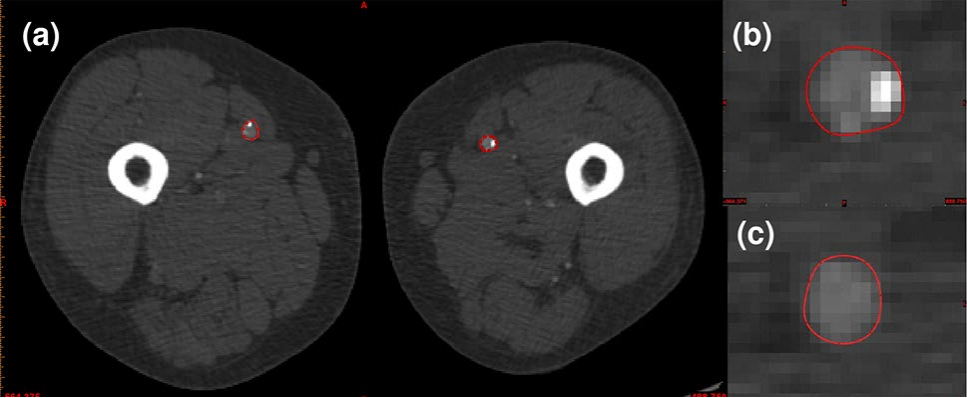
CT scans of the patient’s lower limb in axial view. (a) A CT scan of the patient’s lower limb. (b) A profile of the left femoral artery lumen with calcified plaque, marked by a red line. (c) A profile of the left femoral artery lumen without plaque, marked by a red line
